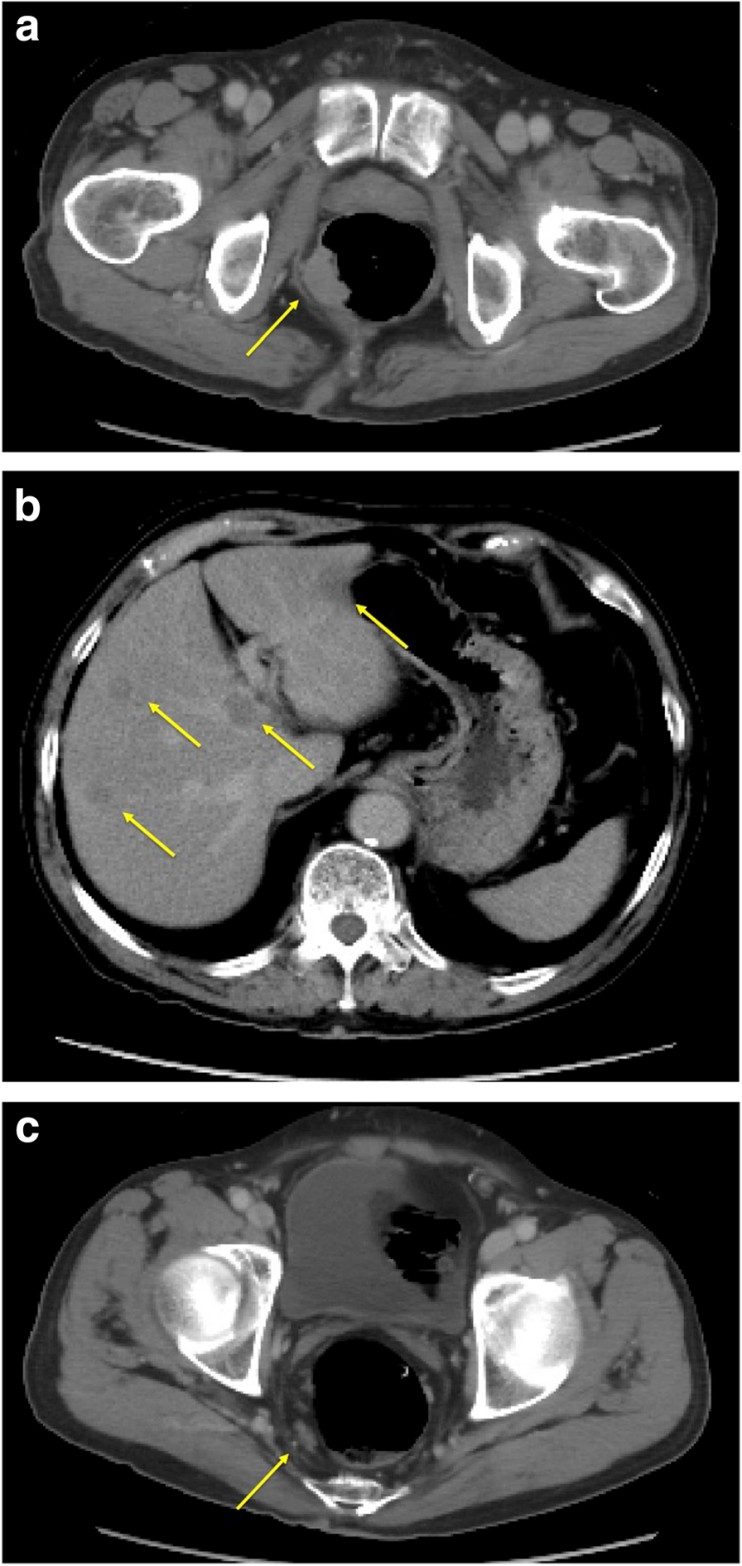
Computed tomography (colonography) image. a This reveals a tumor in the lower rectum with suspected invasion of the right levator ani muscle. b, c The paraintestinal lymph nodes are swollen, and there are multiple liver metastases
